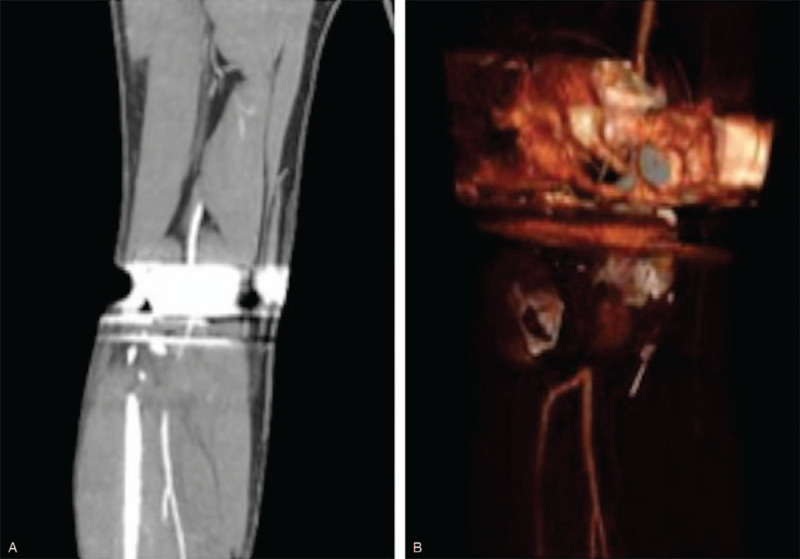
5A-B: Enlarged CT-A images demonstrating clot.
